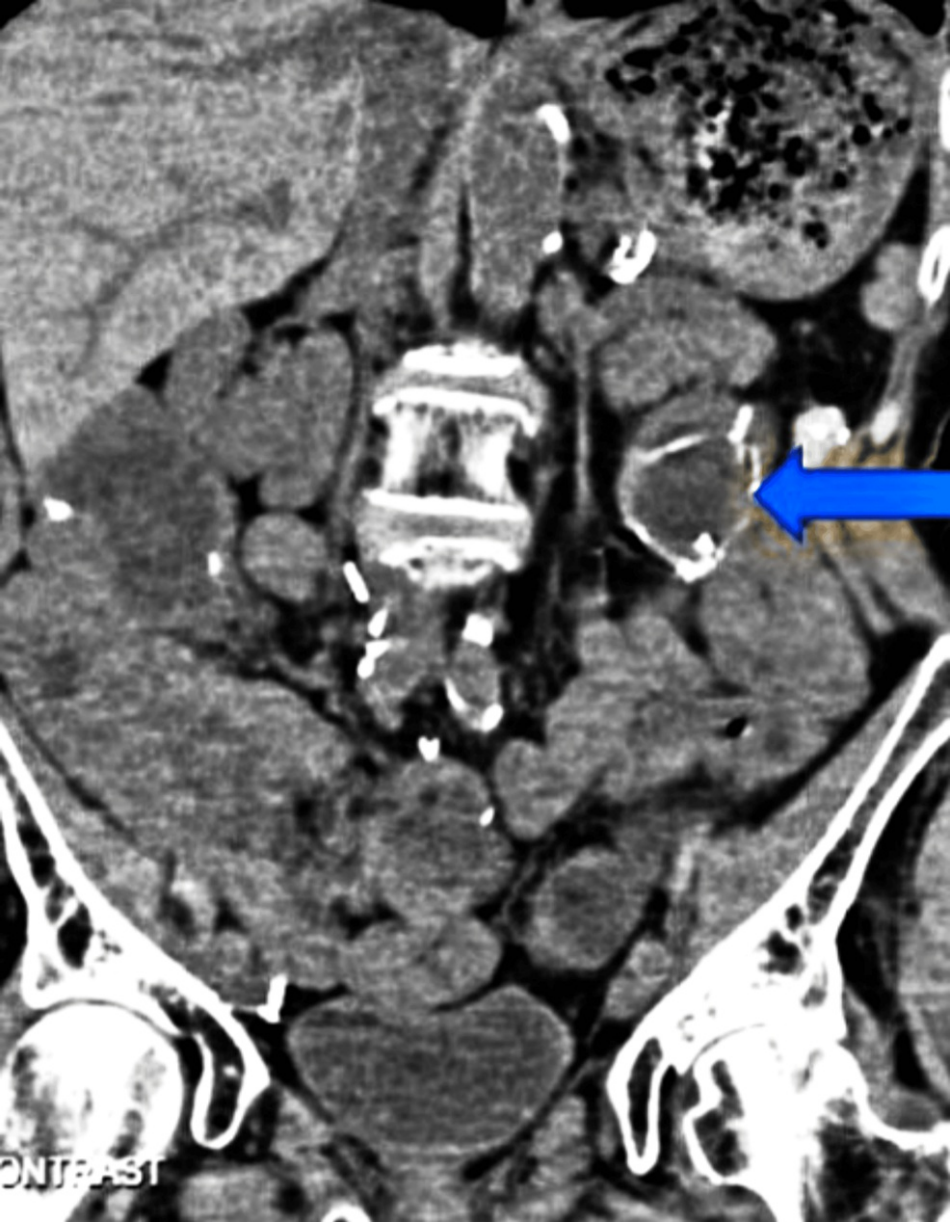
Contrast-enhanced computed tomography abdomen, coronal section with ileostomy (blue arrow)
